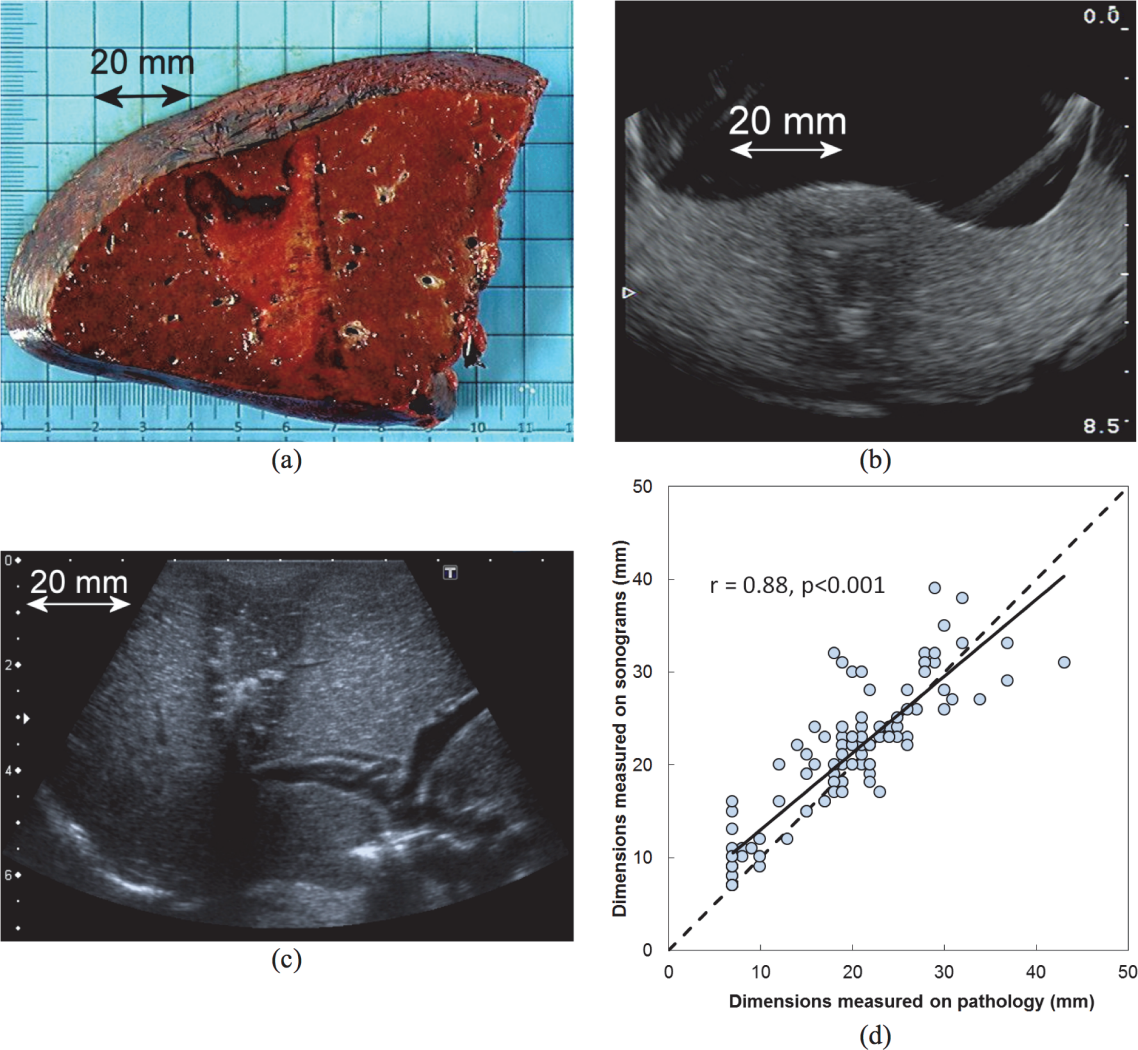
Macroscopic and echographic visualzation of HIFU ablated area. HIFU ablations were clearly visible in ultrasound images. Dimensions measured on sonograms were correlated to the dimensions measured on gross pathology. (a) Typical HIFU ablation observed on gross pathology, (b) on sonograms obtained with the integrated ultrasound imaging probe (IUSP) of the HIFU device and (c) on sonograms obtained with a higher resolution ultrasound imaging probe. (d) Correlation between dimensions measured on sonograms using IUSP and gross pathology.
